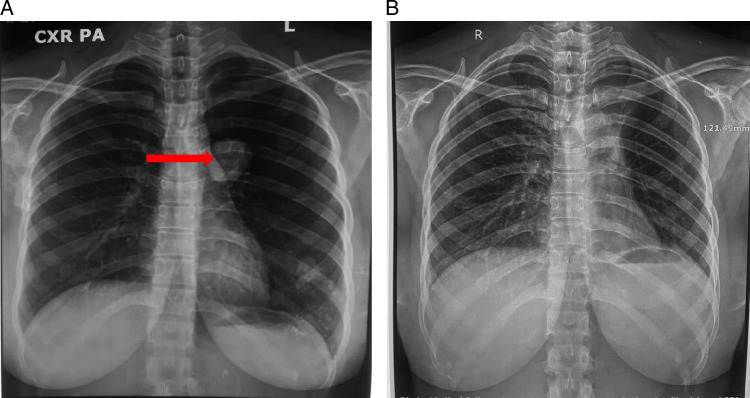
(A) Pre-operative radiograph showing a well-circumscribed solitary nodule in upper left lobe of lung. (B) Post-operative radiograph showing no traces of the nodule in upper left lobe of lung.
